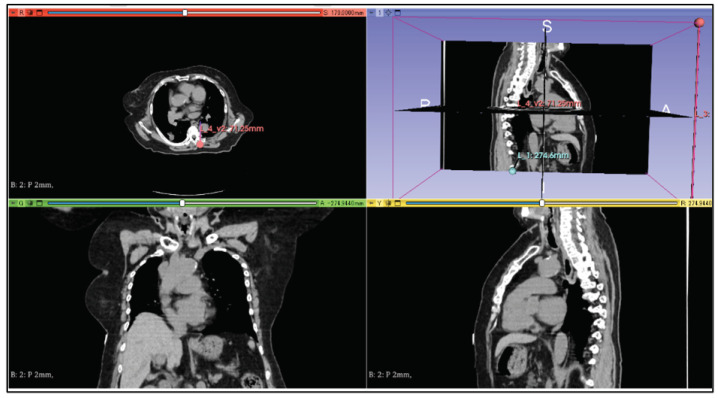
Example of a CT image using 3D Slicer.
